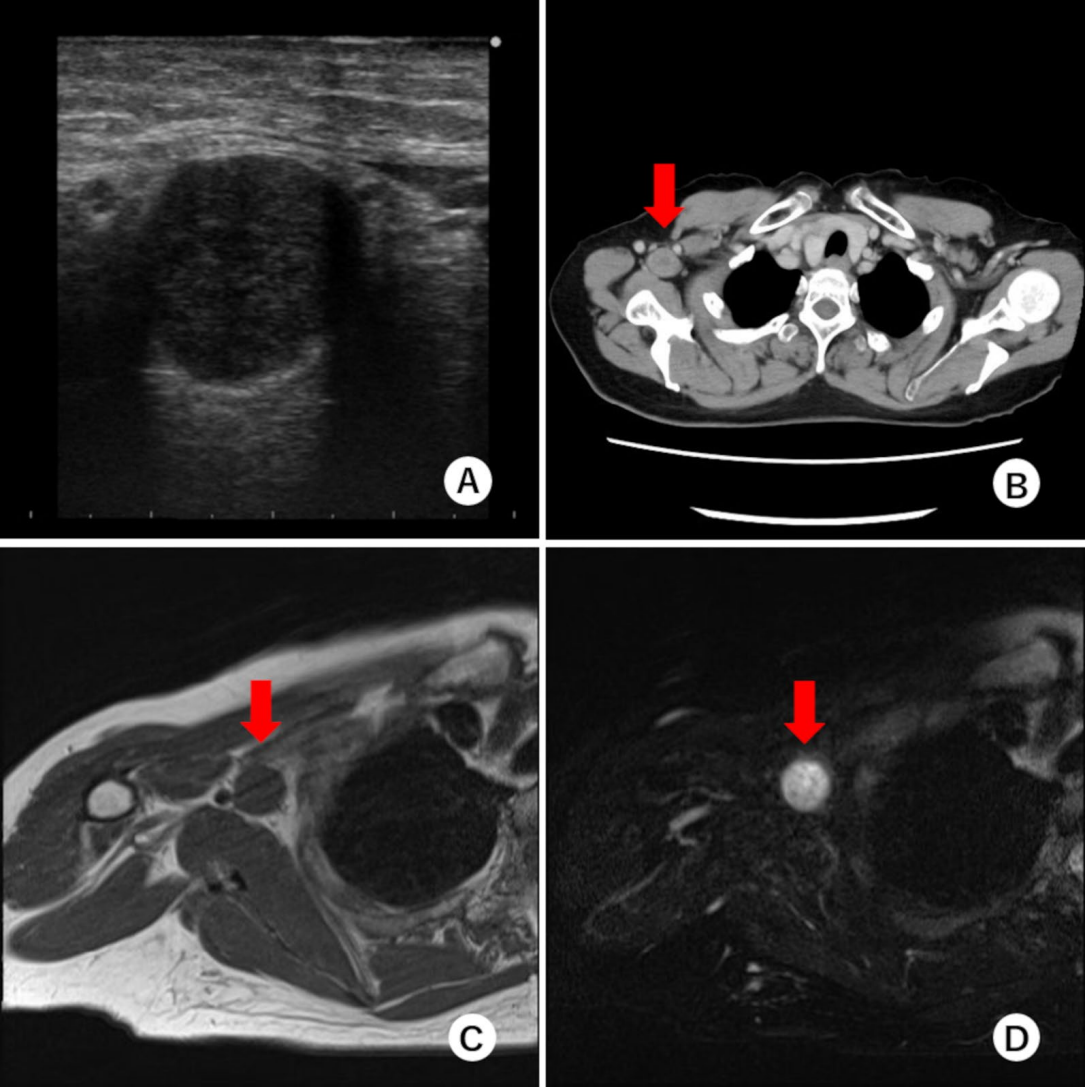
Imaging studies. A Ultrasonography revealed a well-defined hypoechoic tumor in the right axilla region; B enhanced computed tomography revealed a well-defined round tumor in the right axilla region (red arrow). The tumor was in contact with the axillary vein; C, D magnetic resonance imaging revealed a round tumor which had low intensity on T1-weighted images and high intensity on fat-saturation T2-weighted images in the right axilla region (red arrow)
